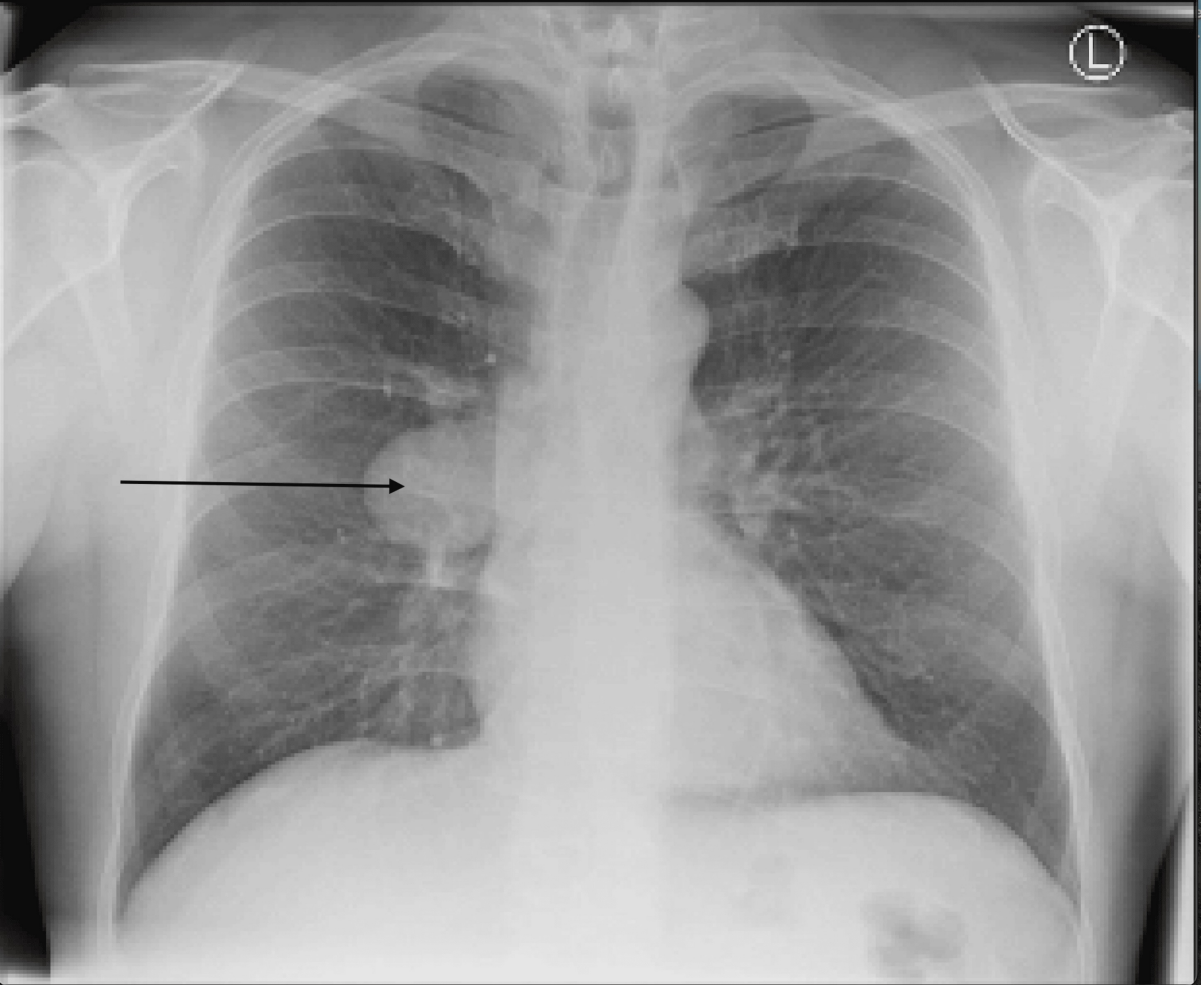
Chest radiograph in PA view, erect, showing a well-defined rounded density overlying the right hilum medially (black arrow). PA: posteroanterior view.
